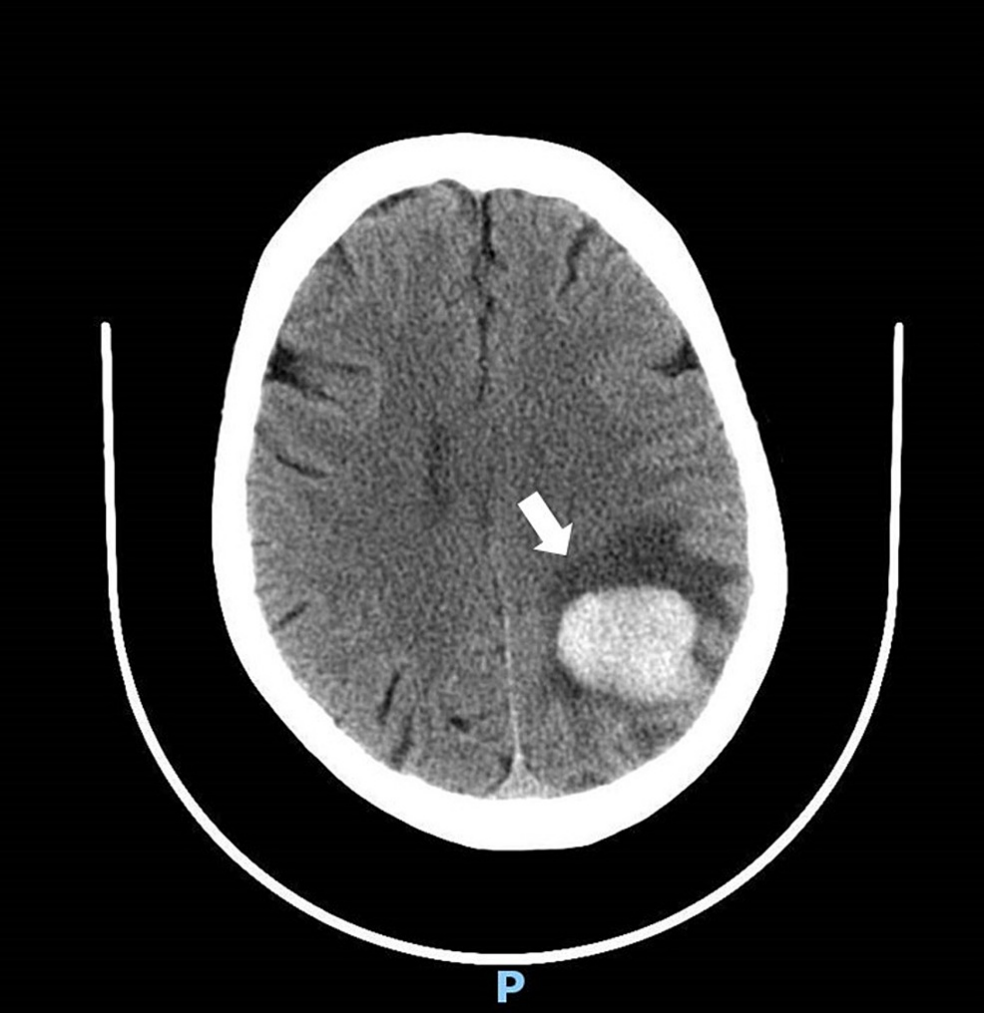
Cranial CT scan showing an acute intracerebral haemorrhage with vasogenic edema
